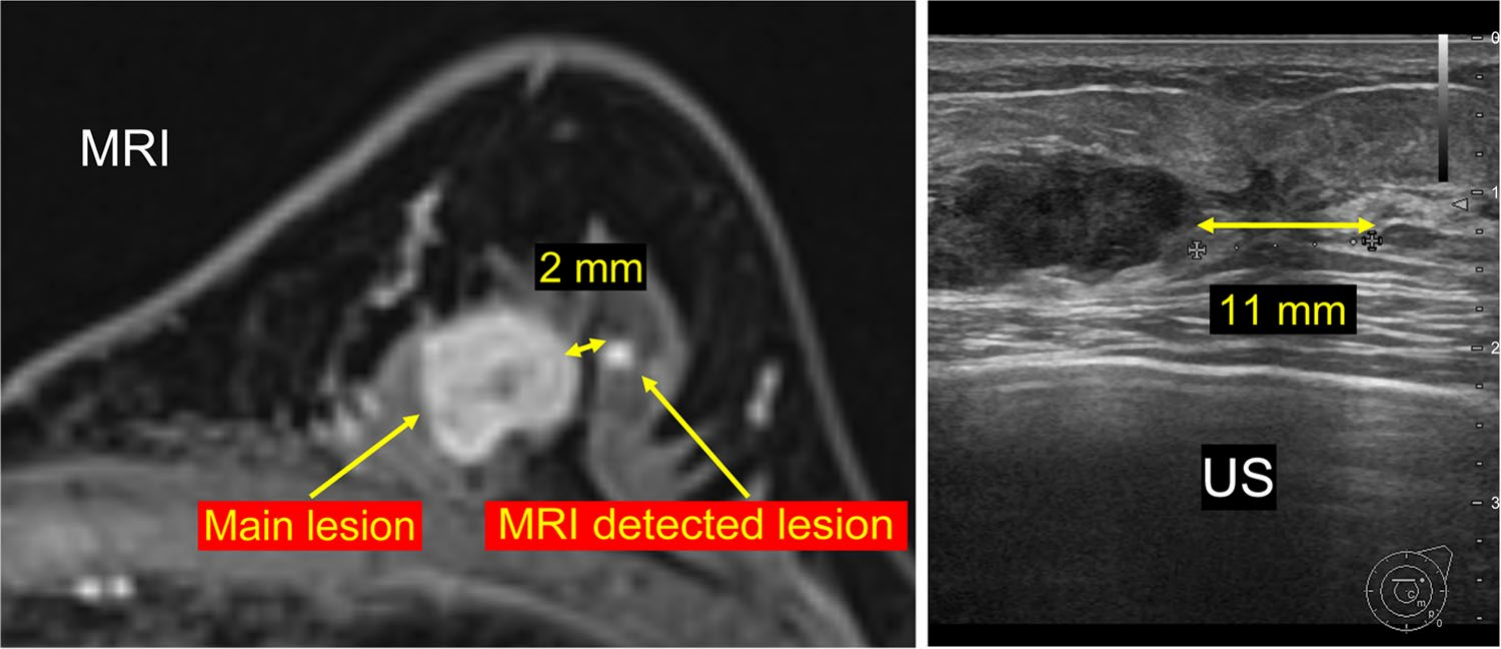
Differences in the spatial relationship of lesions according to patient position. As MRI and ultrasound scans are performed with the patient in different positions (prone and supine, respectively), the spatial relationship of lesions varies between the two imaging modalities. In this figure, the lesions are 2 mm apart on the MRI, but 11 mm apart on the ultrasound image. Owing to such differences, identifying MRI-detected lesions using ultrasound alone is difficult
